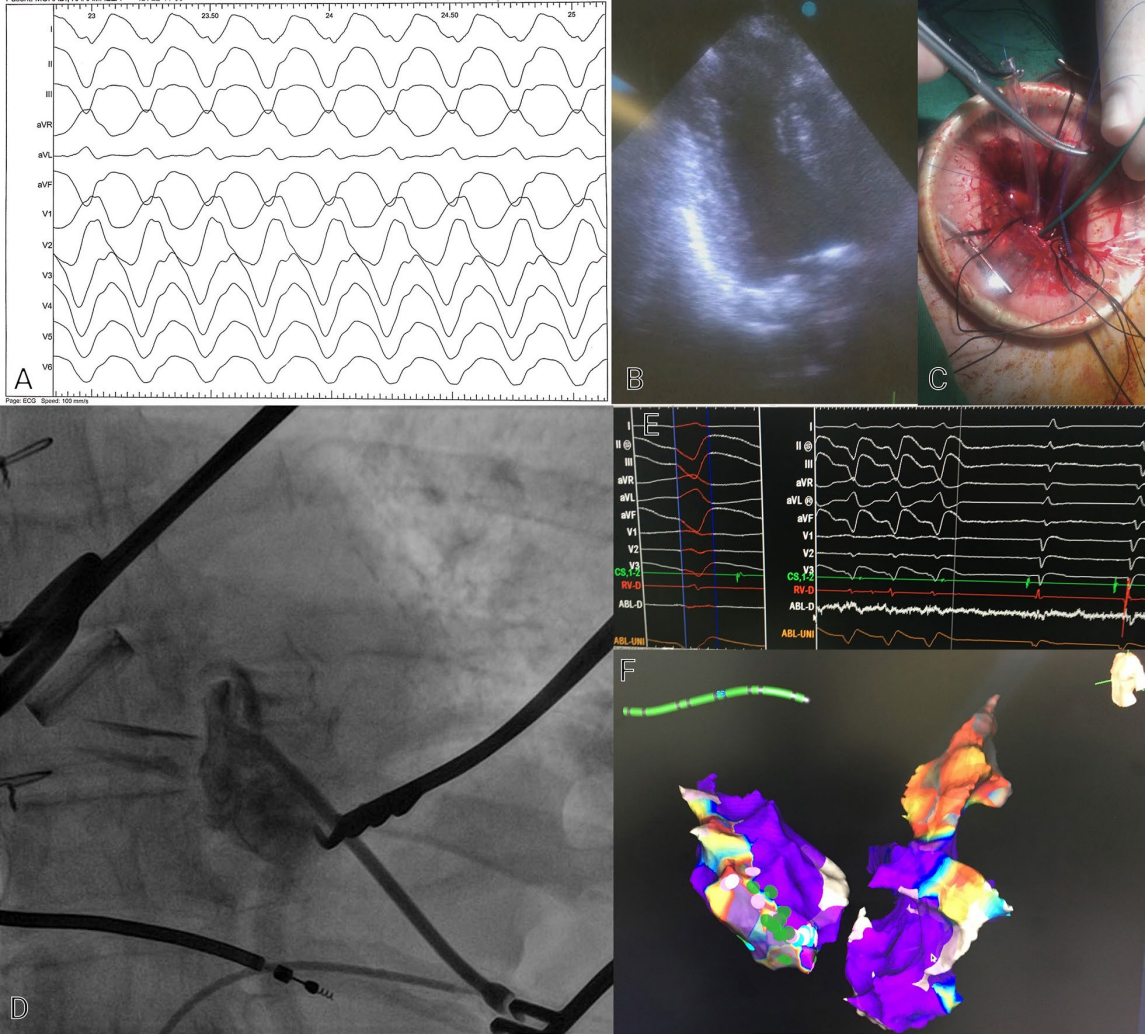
EPS, transapical access and mapping of ventricular tachycardia, and RF ablation. EPS was done and showed easily inducible VT, originated mainly from posterior aspect of LV apex A. Echocardiography showed aneurysmal LV apex B and the surgeon made an incision in the left thorax with the guide of echocardiography at LV apex C and inserted the ablation catheter using the modified Seldinger approach. Left ventriculography was done to prevent inadvertent contact between the ablation catheter and the metallic prosthetic valves D. RF ablation was done and VT was terminated E. Voltage mapping of LV aneurysm and epicardium along with RF ablation spots
